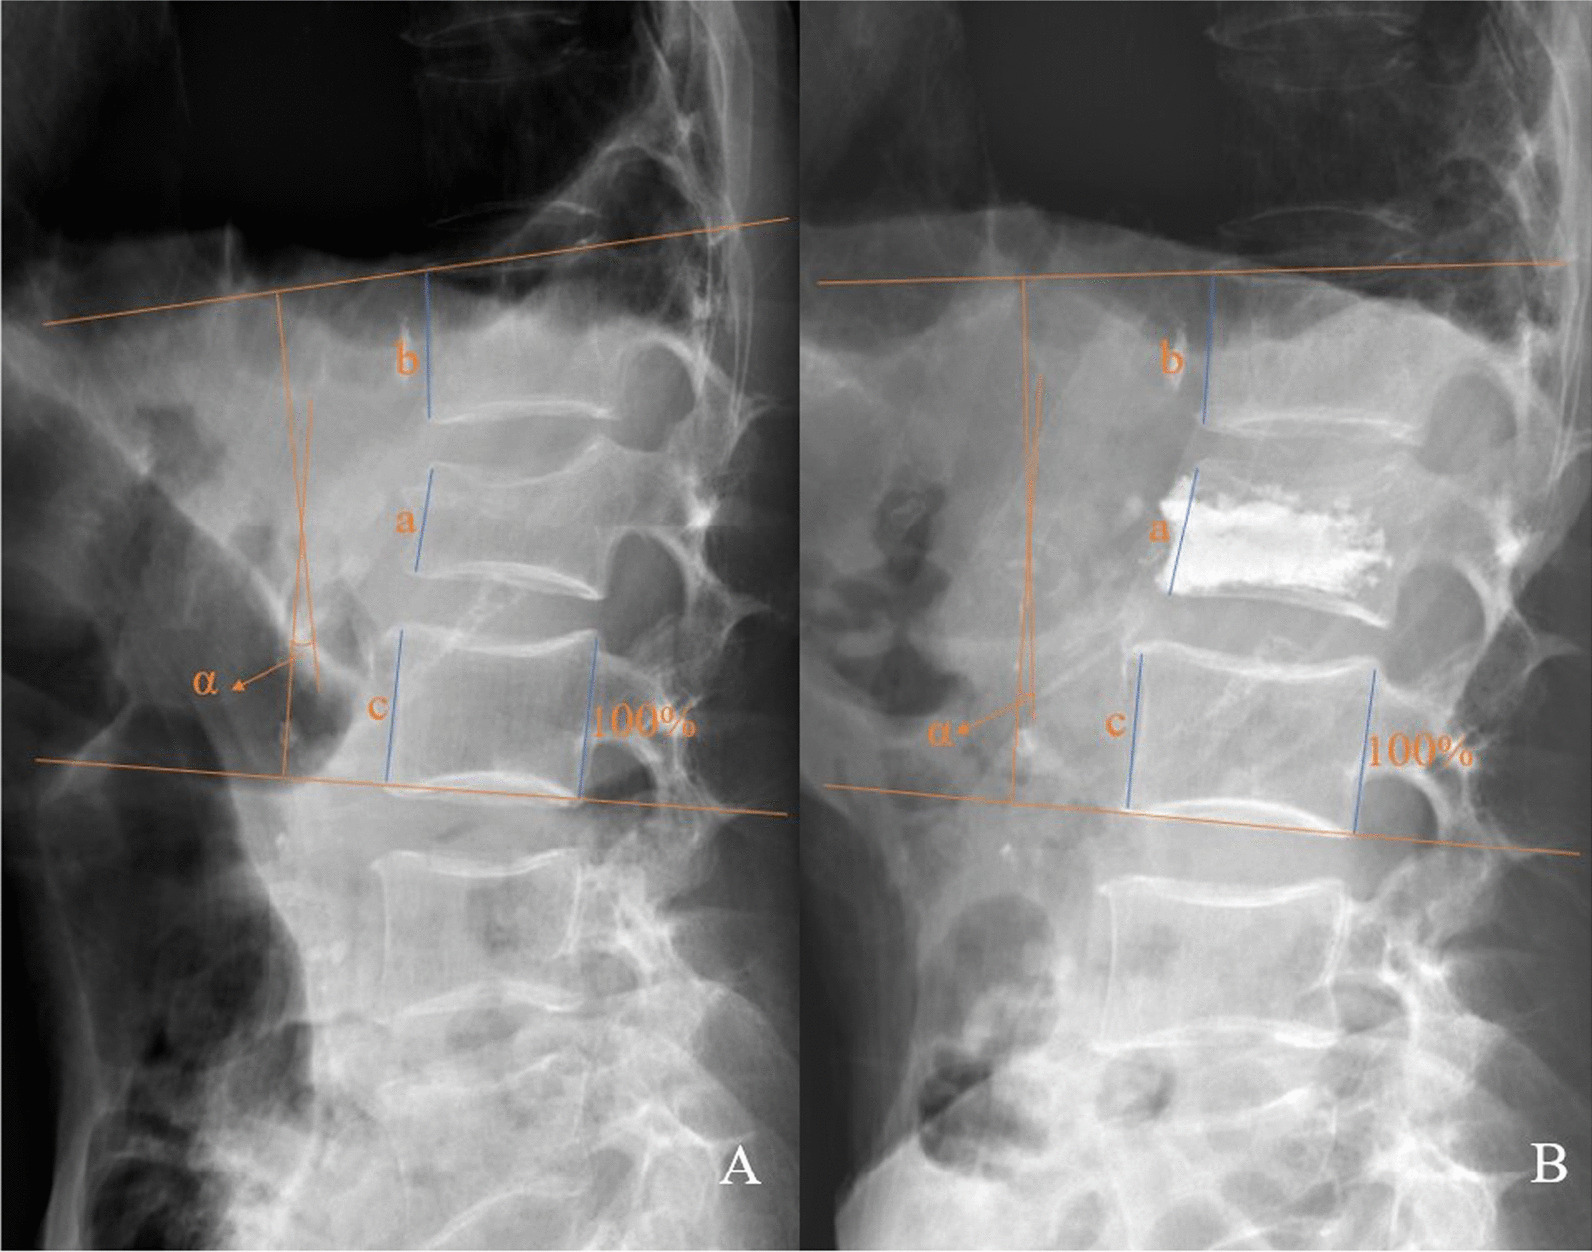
Relative anterior height (RAH) of the fractured vertebra and the kyphotic angle measurement method. Measurement of anterior height and kyphotic angle before (A) and after (B) operation. The posterior height (PH) of caudal healthy vertebra, which was adjacent to OVCF, was measured and transferred as 100% on the radiograph. TRA = *a*/[(*b* + *c*)/2]. The kyphotic angle (α) was measured as the angle between the superior endplate at one level above the injured vertebrae and inferior endplate at one level below the injured vertebrae
